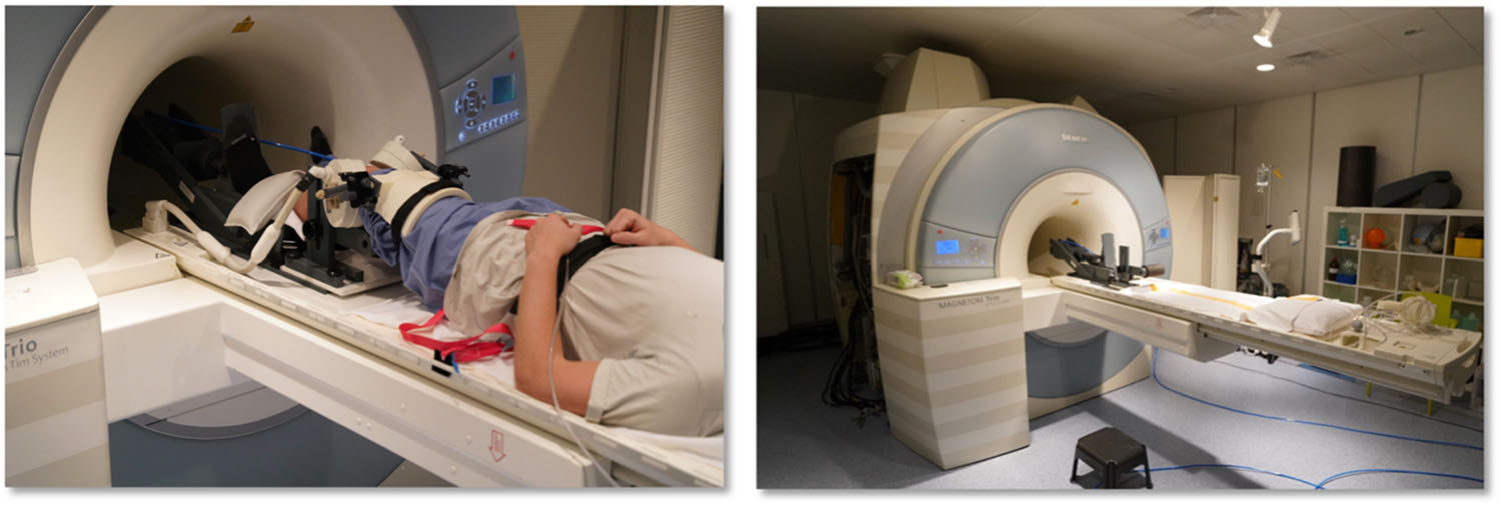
Experimental setup for the MRI examinations (left) with a pneumatic loading device (right). MRI, magnetic resonance imaging.
